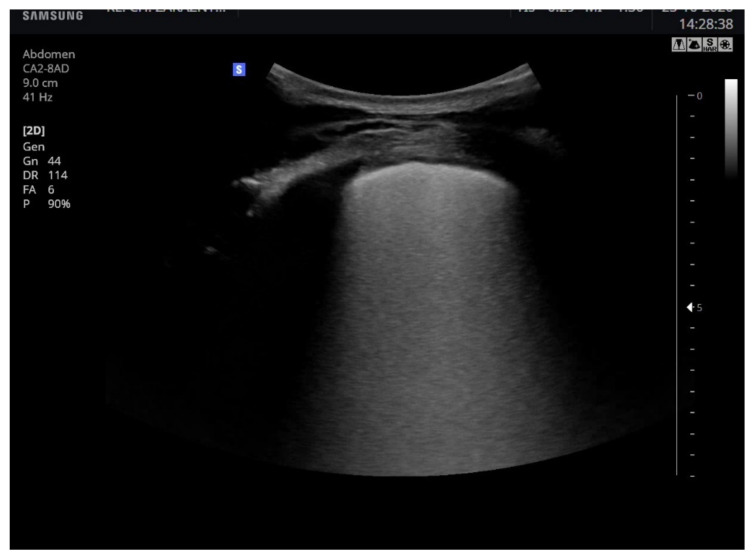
Lung ultrasound demonstrating areas of “white lung”.
